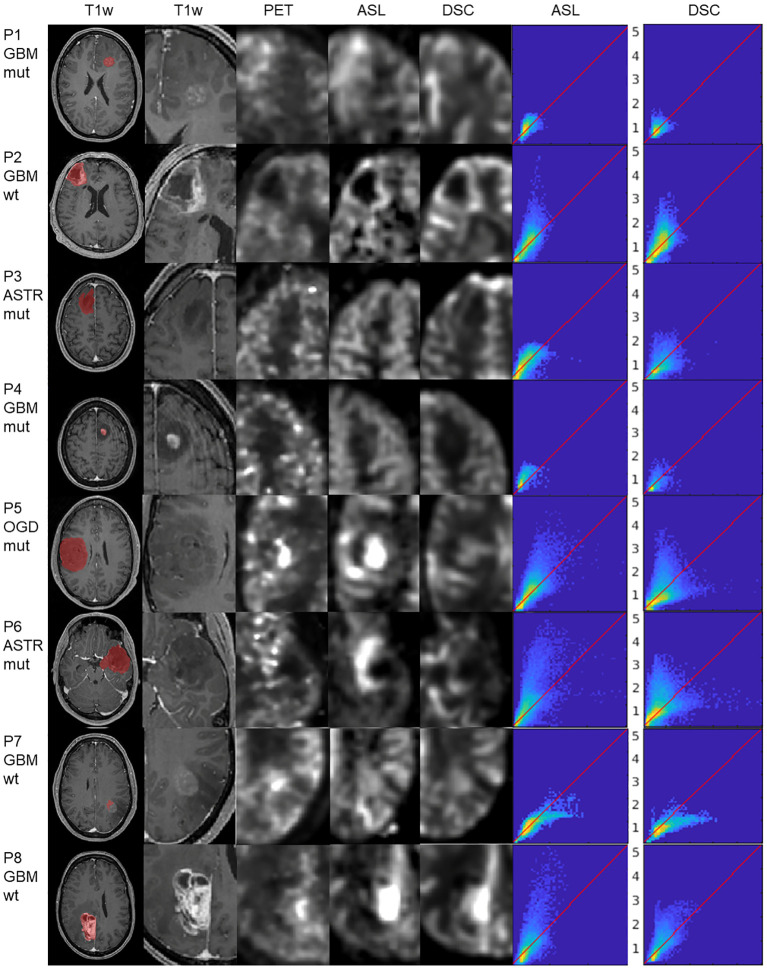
The figure shows a representative slice from each participant, including the tumor. The first column shows the T1-weighted whole-brain image in native resolution with the tumor ROI highlighted in red. The following columns show cropped regions including the tumor T1 for the following sequences: T1-weighted post gadolinium in native resolution; PET-CBF, ASL-CBF, and DSC-CBF, all smoothed to PET native resolution; and joint histograms of CBF_GMnorm_ over the tumor ROI comparing ASL-PET and DSC-PET (the scale of the histograms is 0–5 representing the normalized CBF values). ASL, arterial spin labeling; CBF, cerebral blood flow; DSC, dynamic susceptibility contrast; GBM; glioblastoma; GM, gray matter; ROI, region of interest; WM, white matter.
